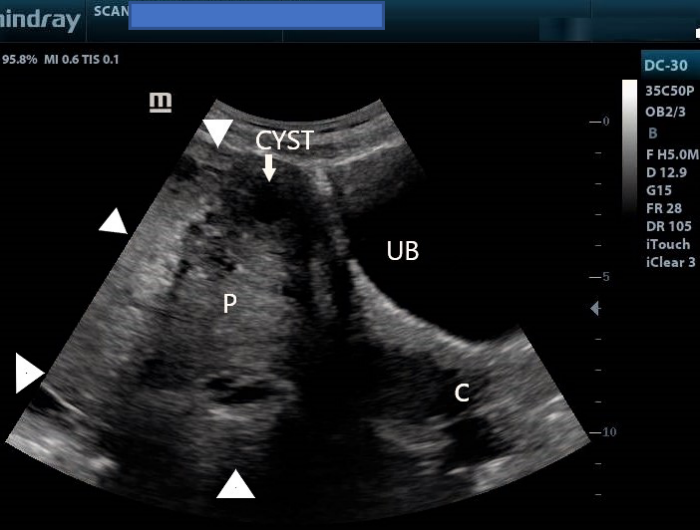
Lower uterine segment ultrasound image showing the urinary bladder and internal cervical os (C) completely covered by the placenta from anterior to posterior. White arrowheads indicate placental margins. Note also the anterior placental cyst. UB refers to the urinary bladder
